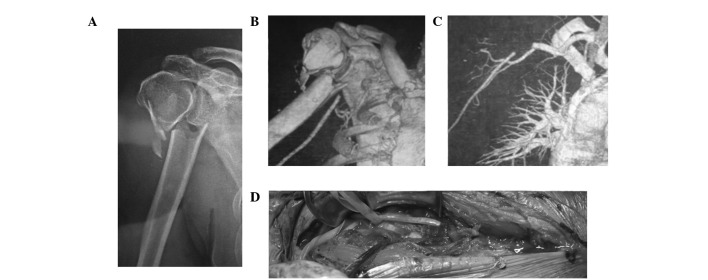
Axillary injury of case 2. (A) Radiograph of the right proximal humerus with a comminuted fracture of the surgical neck. (B) CTA shows the association of the injured artery and the bony segment. (C) CTA shows the thrombosed axillary artery. (D) Intraoperative photograph of the thrombosed axillary artery. CTA, computerized tomography angiography.
